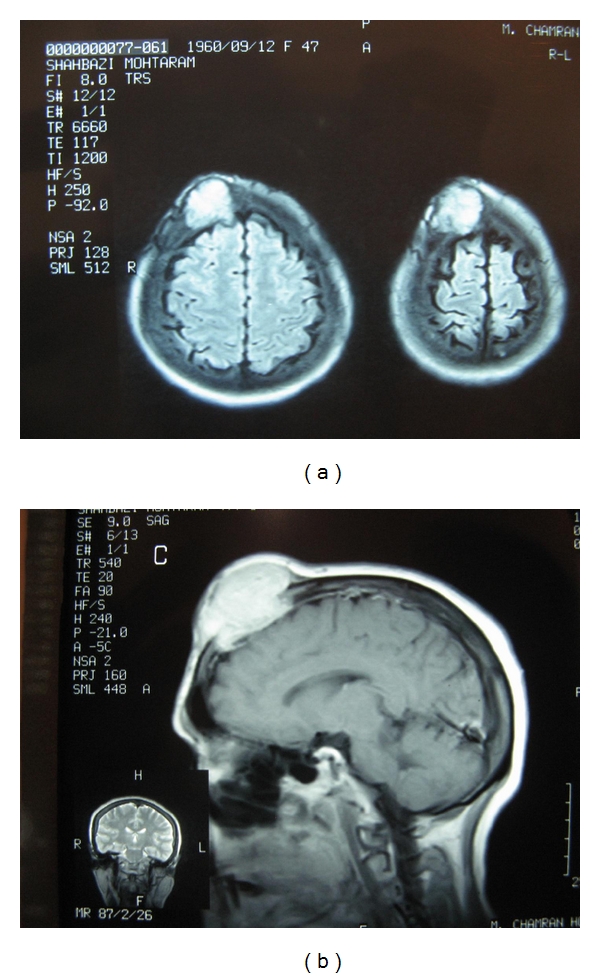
Magnetic resonance image revealed a large destructive mass at right frontal bone with soft tissue invasion.
